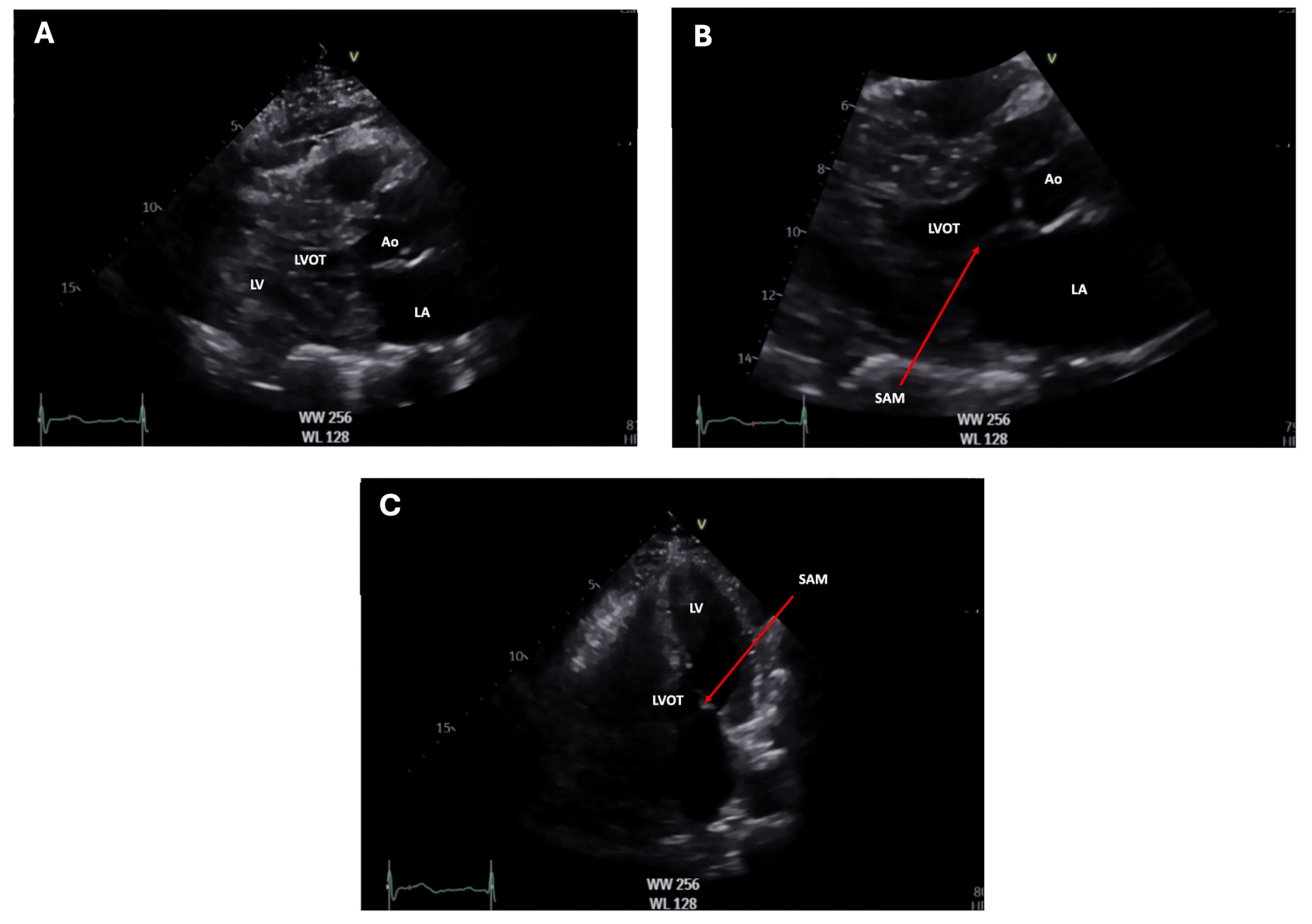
Transthoracic echocardiography (TTE) views of the patient before liver transplantation. Image A demonstrates a parasternal long axis (PLAX) view during late systole. Image B shows the PLAX view with systolic anterior motion (SAM) of the mitral valve during late systole. Image C shows the apical five-chamber view during mid-systole.
